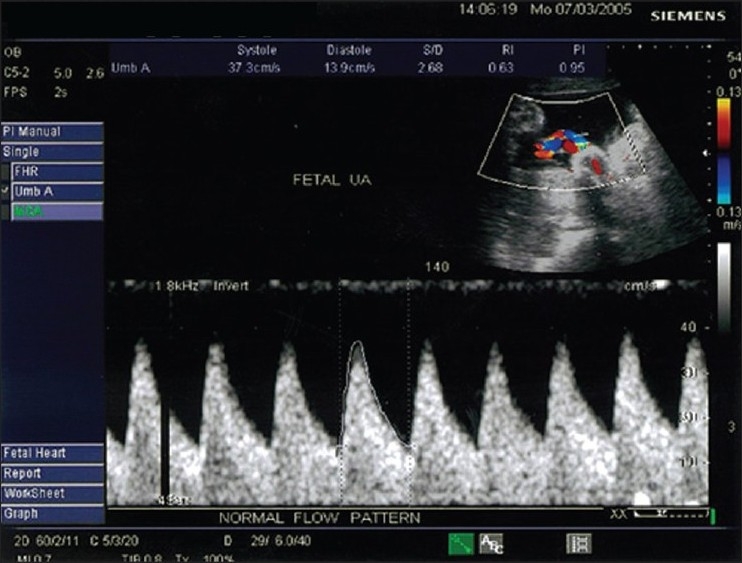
Color Doppler of the umbilical artery shows a normal UA waveform pattern with low impedance, high diastolic flow and decreased pulsatility index
